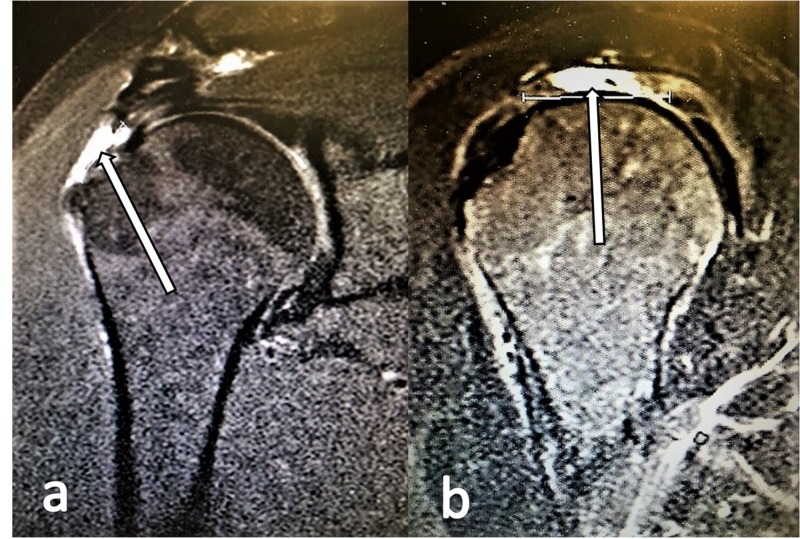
Supraspinatus tears depicted in MRI scans. a. T2-weighted oblique coronal MRI view with a high-intensity signal (white arrow) revealing a full-thickness tear of the supraspinatus. b. T2-weighted oblique sagittal MRI view with a high-intensity signal (white arrow) revealing a full-thickness tear of the supraspinatus.
